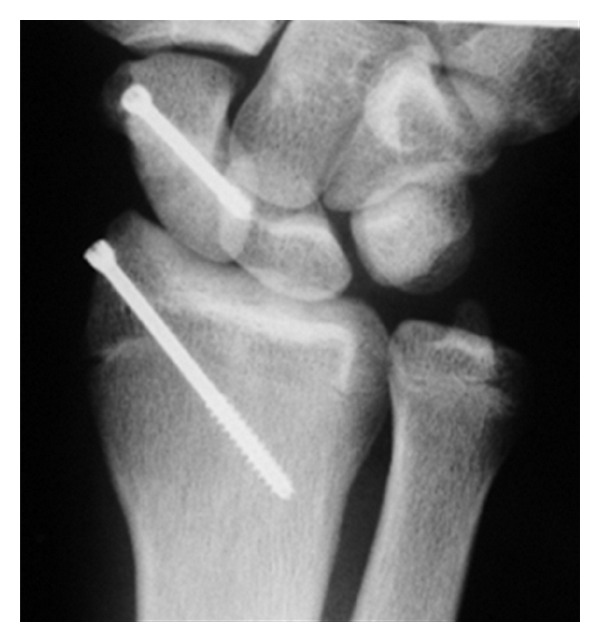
Plain PA radiograph shows the percutaneous fixation and radial styloid process in situ fixation 8 weeks after operation.
